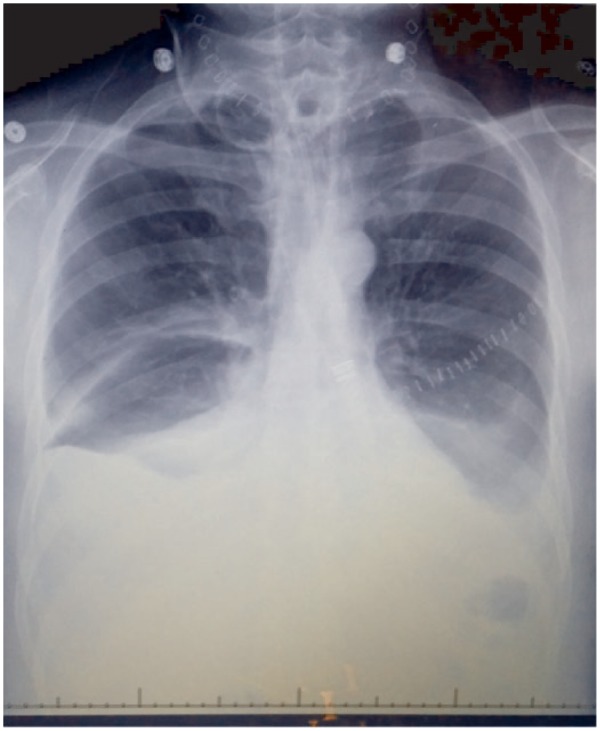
Chest X-ray 1 week after the surgery, interpreted as bilateral basilar atelectasis. Staples over the left thorax are a result of left pectoralis major pedicled myocutaneous flap reconstruction.
